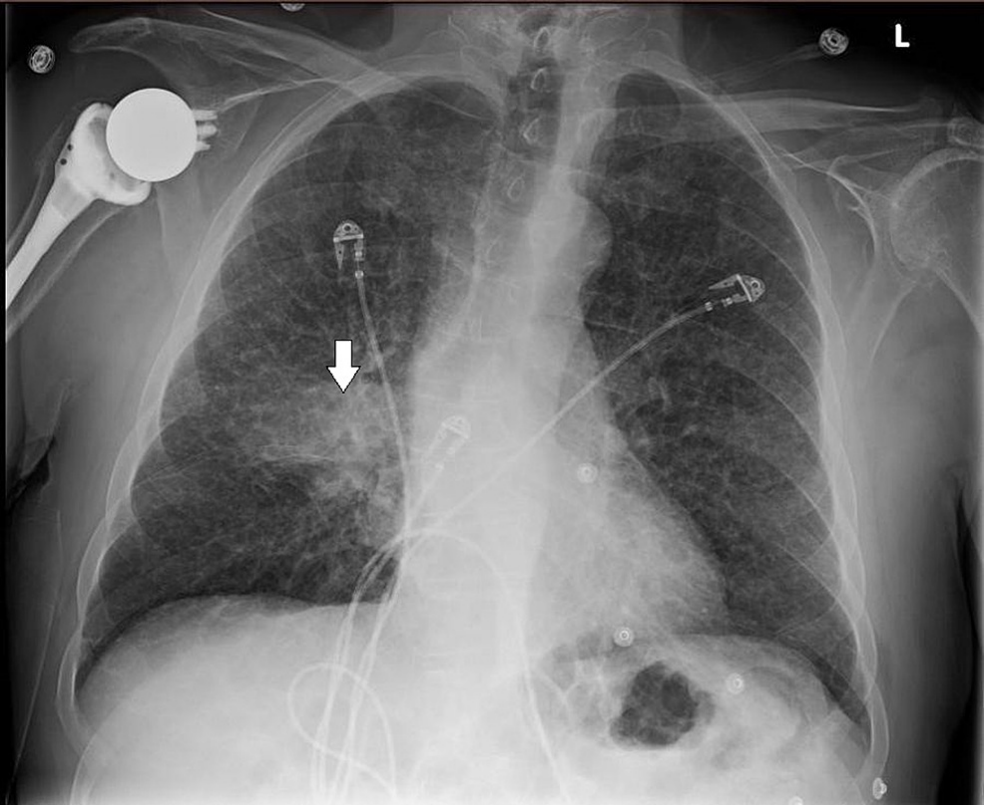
Chest x-ray showing dense right lower lobe infiltrate (arrow)
